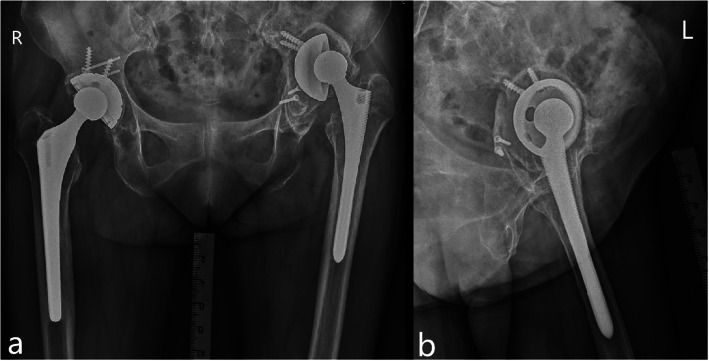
Preoperative anteroposterior (a) and lateral (b) radiograph show a 74-year-old female presented with Paprosky IIIa acetabular defects after primary THA
